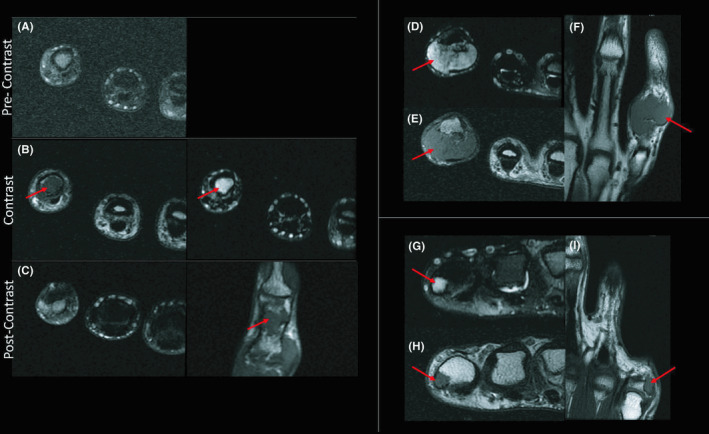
Original MRI showing an enhancing lesion with distinct areas of T2 hyperintensity and T1 hypointensity (B, C: arrows) separated by regions of T1 hyperintensity. MRI showing a T2 hyperintense and T1 hypointense (G‐I: arrows) lesion measuring 0.5 x 0.6 x 0.9 cm on the ulnar base of the 5th proximal phalanx
